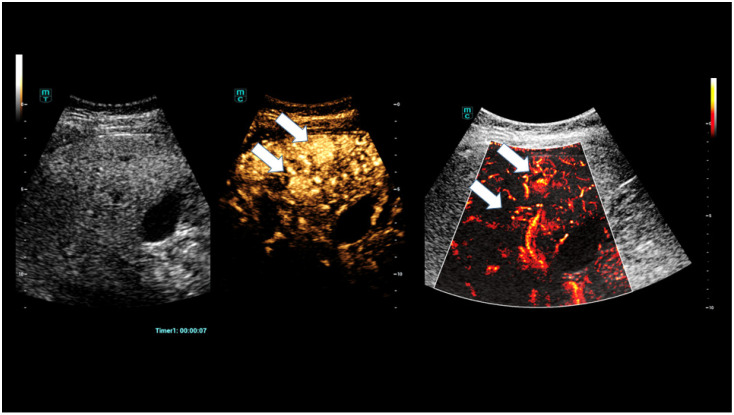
CEUS and SR CEUS after injection of 1.4 mL ultrasound contrast agent with irregular hyperenhancement of the liver and early nodular enhancement of high-flow haemangiomas (arrows) and micro-shunts.
